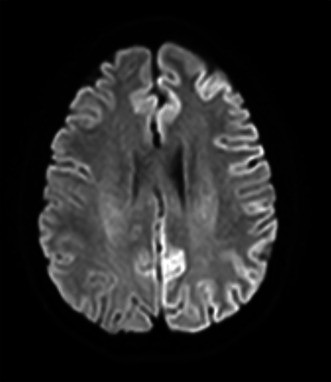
FIGURE 2 Axial diffusion‐weighted MRI showing cortical ribboning in the frontoparietal regions.
